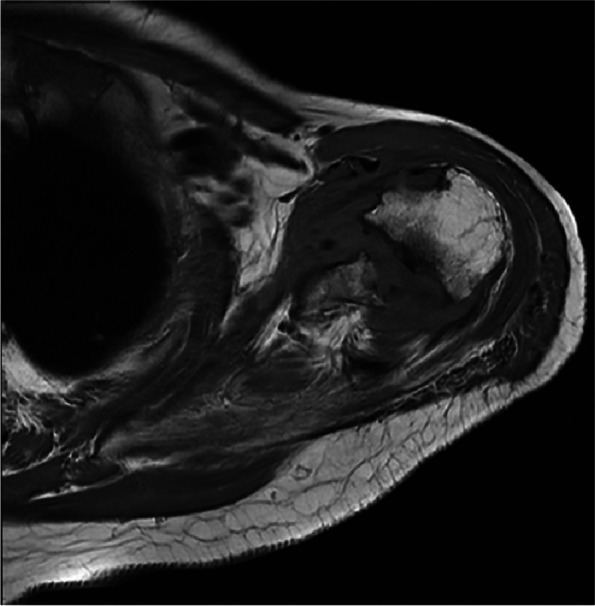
Transverse MRI of the left shoulder joint
